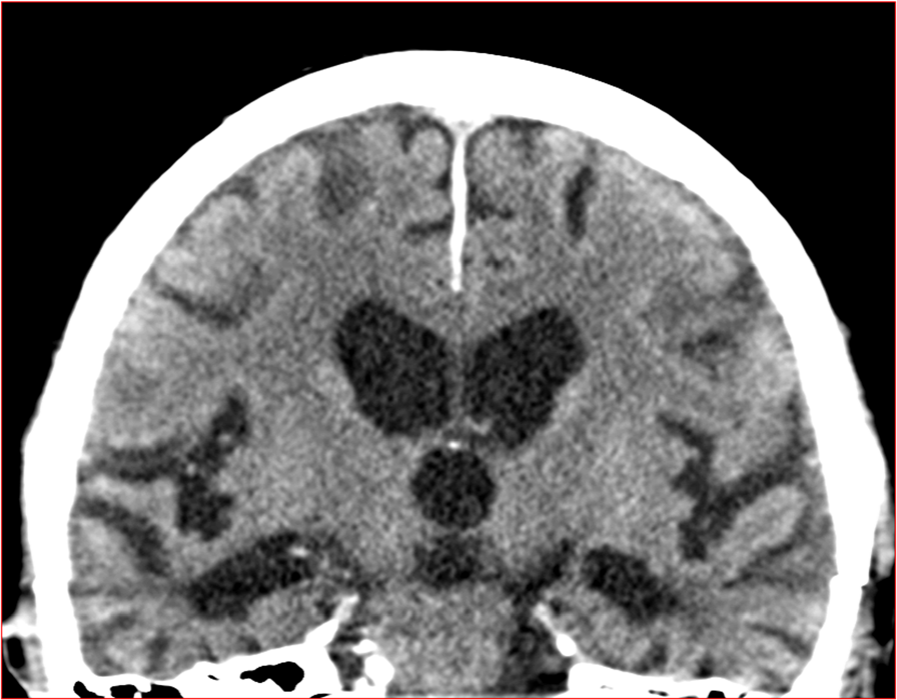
Medial temporal lobe atrophy. Example of abnormal medial temporal lobe atrophy in a CT scan in a study patient representing a score of 3 on the left side and 4 on the right side. This was not mentioned in the original report. This patient had an MMSE score of 22 points with 0 points on the memory item. This patient had noprevious mentioning of cognitive impairment in medical records
